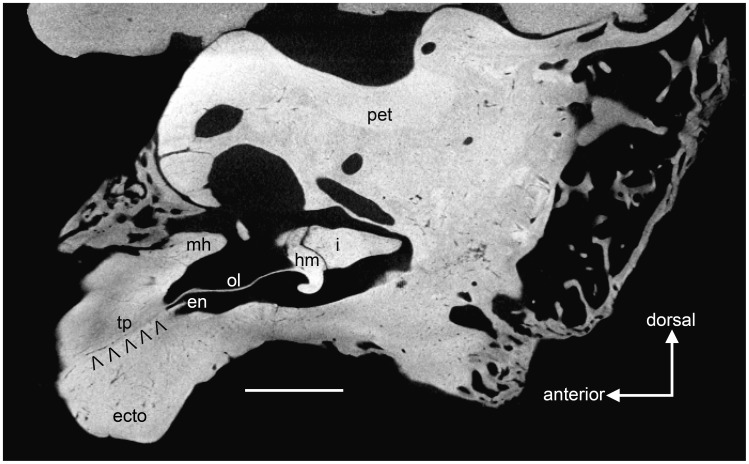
Felis catus*, adult, longitudinal section through the middle ear generated by Avizo®7 from CT data [42] (see text for methods). Seam between tympanic plate of mallear rostral process and ectotympanic is marked by carets. Scale = 2 mm. Abbreviations: ecto, ectotympanic; en, ectotympanic notch; hm, head of malleus; i, incus; mh, mallear hook; ol, osseous lamina; pet, petrosal; tp, tympanic plate of rostral process.
